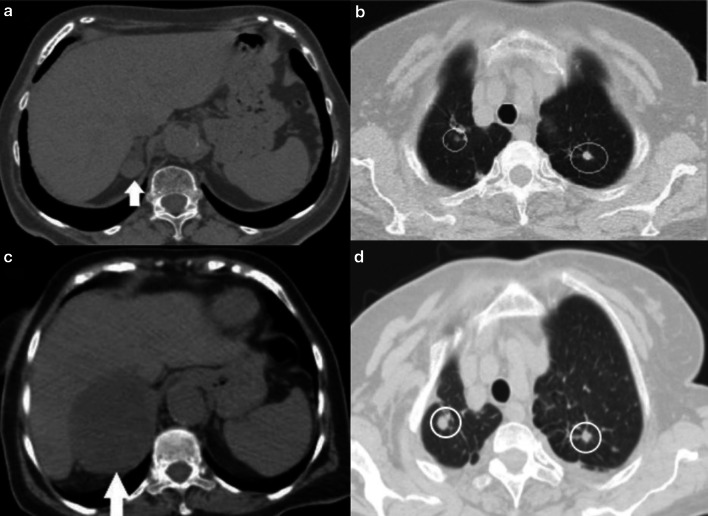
Hyperprogression, a 74-year old female patient with metastatic non-small cell lung cancer under treatment with atezolizumab (anti-PD-L1 monoclonal antibody). a, b Before the initiation of immunotherapy, axial CT images show the metastases in the right adrenal gland (arrow) and upper lobes of both lungs (circles). c Axial CT image shows an increase in tumor burden of target lesion more than 50% compared with scans done before immunotherapy initiation. d There is also an increase in size in non-target lesions in both upper lung lobes(circles)
